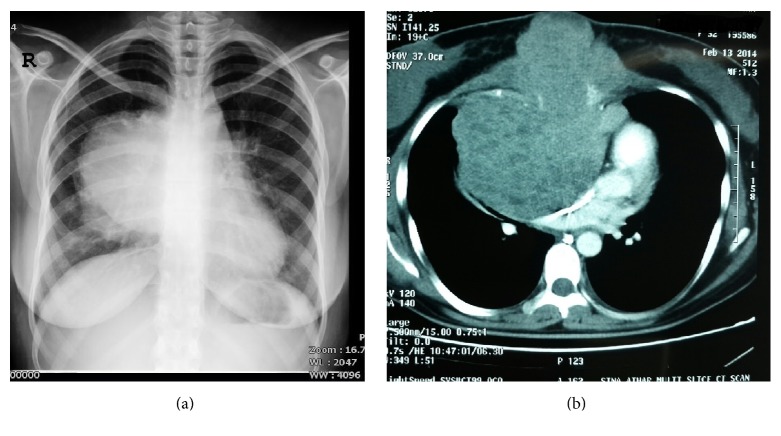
Preoperative chest X-ray (a) and CT scan (b) showing a large mass with multiple areas of low attenuation that has compressed the heart but without obvious invasion to vital organs.
